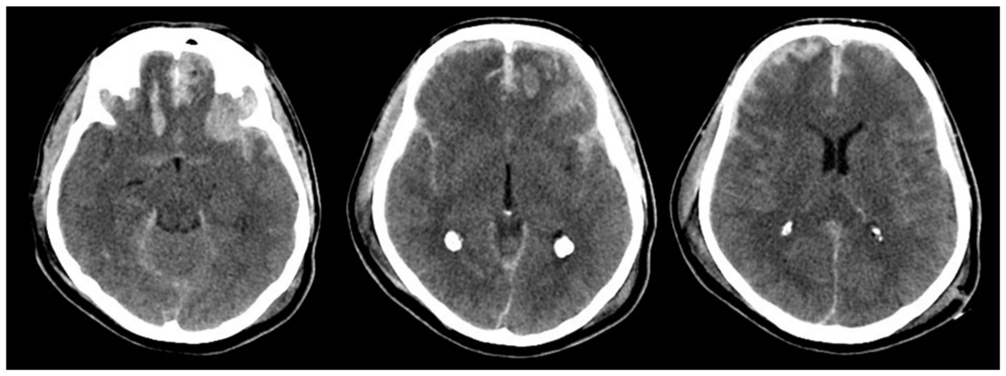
A fifty nine year old male had suffered a fall. Initial Glasgow coma score was recorded as six (Eye: 1, Motor: 4, Verbal response: 1). His right pupil was unreactive. Left pupil was reactive. There were no other injuries. CT scan of the brain revealed diffuse petechial haemorrhages, traumatic subarachnoid haemorrhage and non evacuated haematoma.
